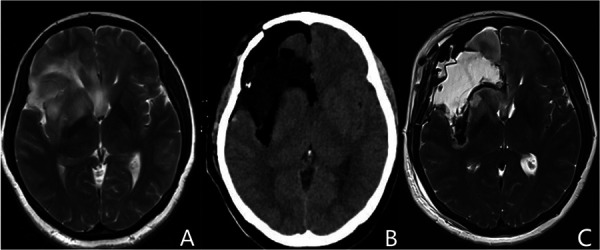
Representative images of the basal ganglia outline reappearance. (A) Preoperative MRI shows that the basal ganglia is invaded by insular gliomas. (B,C) Postoperative CT and MRI T2 imaging demonstrate the artificial profile of the basal ganglia, which is called the basal ganglia outline reappearance. MRI, magnetic resonance imaging; CT, computed tomography.
